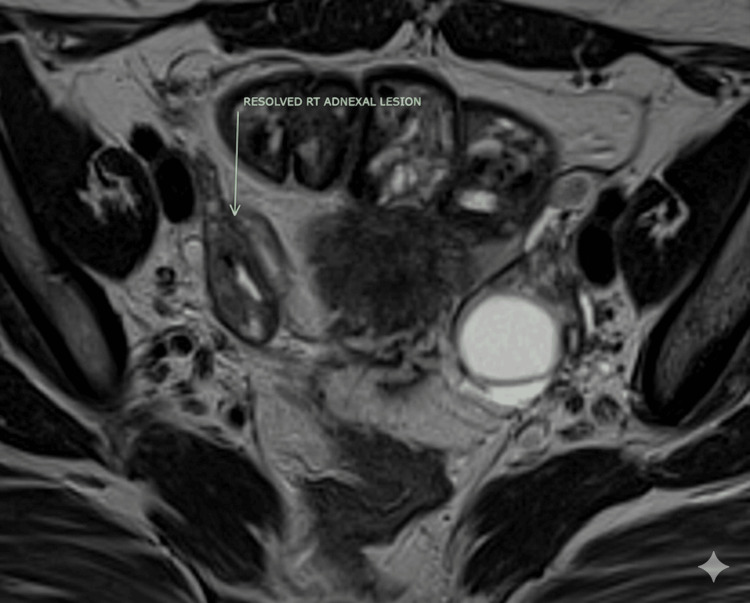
MRI of Case 2 showing the resolved adnexal mass and normal pelvic anatomy
